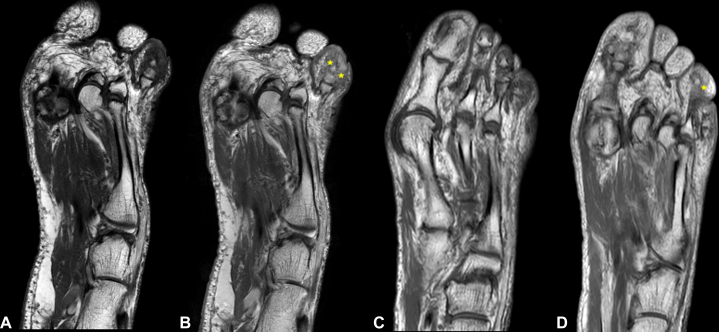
POPP: Follow-up T1-weighted MRI postgadolinium demonstrates decreased contrast enhancement and reduced soft tissue edema surrounding the fourth toe (*asterisks*), comparing baseline (A and B) and 1 year after treatment with ixekizumab (C and D). MRI: pre- (A and C) and post-gadolinium (B and D). *MRI*, Magnetic resonance imaging; *POPP*, psoriatic onycho-pachydermo periostitis.
